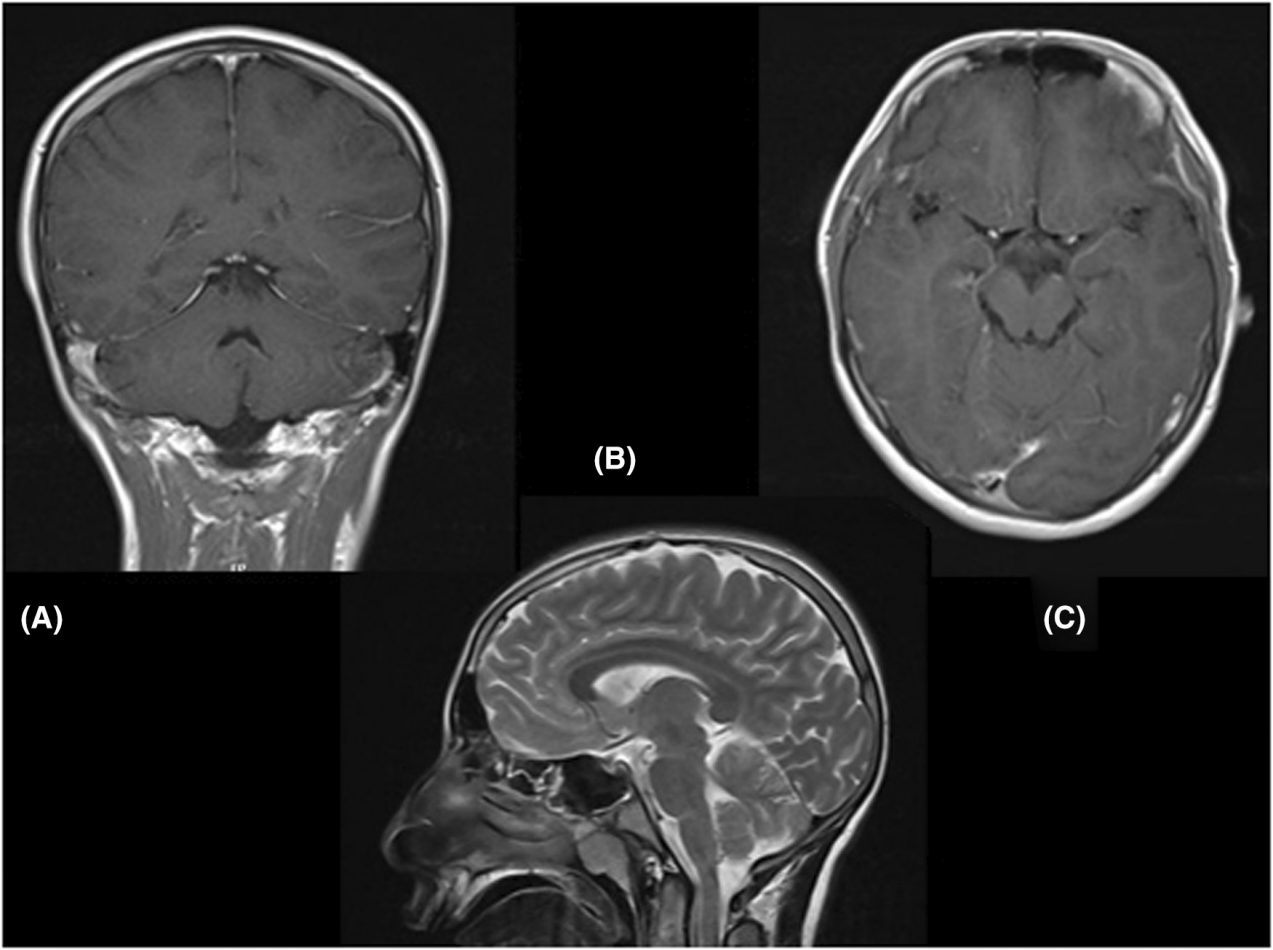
Brain MRI of the patient A: coronal view, B: sagittal T2 view, C: axial T1 view with contrast, showed no abnormalities with or without contrast
